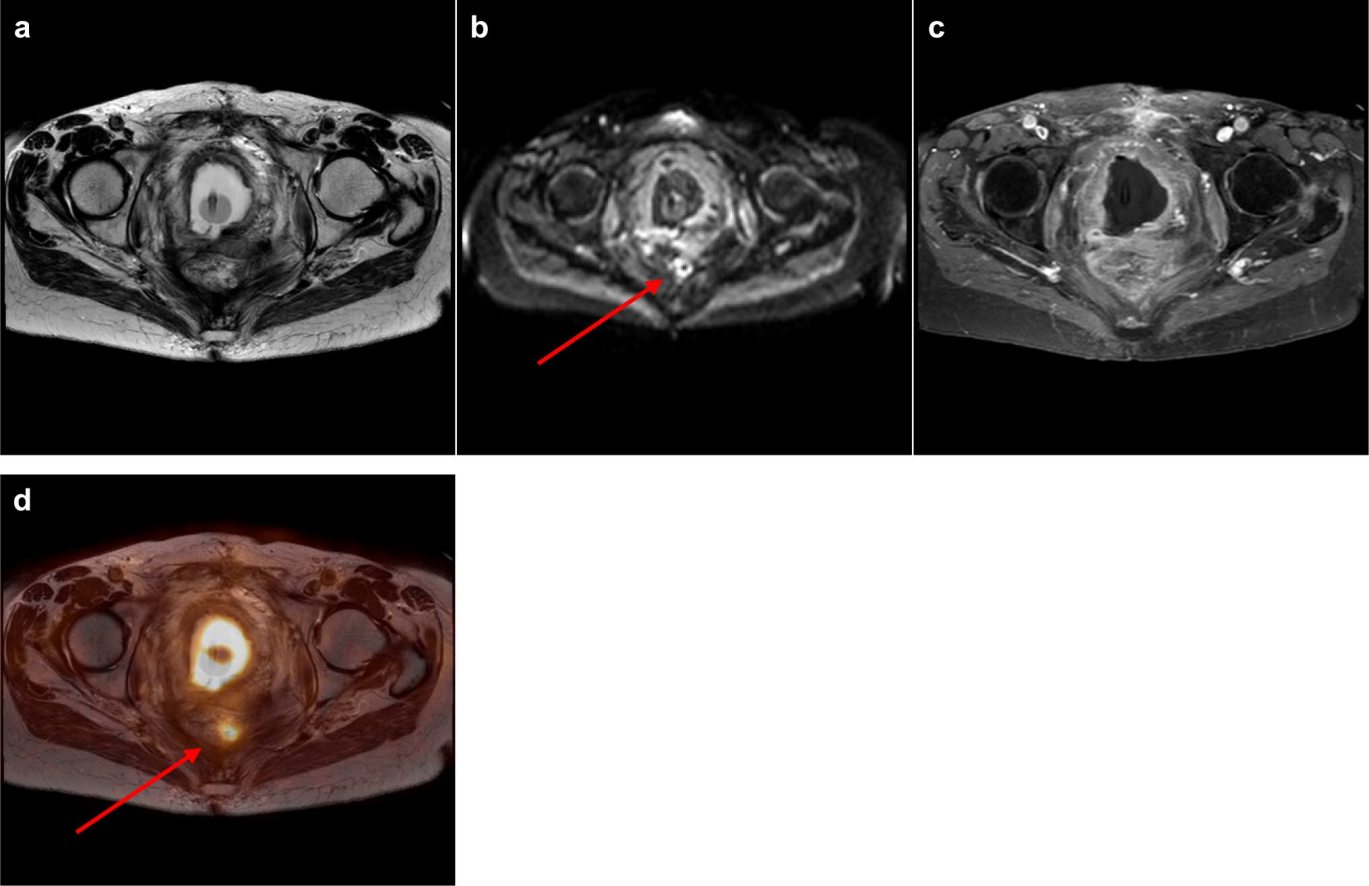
a–d Small presacral tumor recurrence. a–c Axial T2-weighted/axial diffusion-weighted/axial T1-weighted contrast-enhanced MRI. MRI showing diffuse T2-hypointense changes presacrally, a small rim-like diffusion restriction and no focal contrast enhancement. Diffusion restriction was thought to be due to reactive/inflammatory changes. MRI was scored as recurrence unlikely (score 1). d Corresponding axial PET/MRI fusion image demonstrating a small presacral lesion with intense FDG-uptake, which was interpreted as highly suspicious (score 4) for tumor recurrence. Biopsy confirmed tumor recurrence
